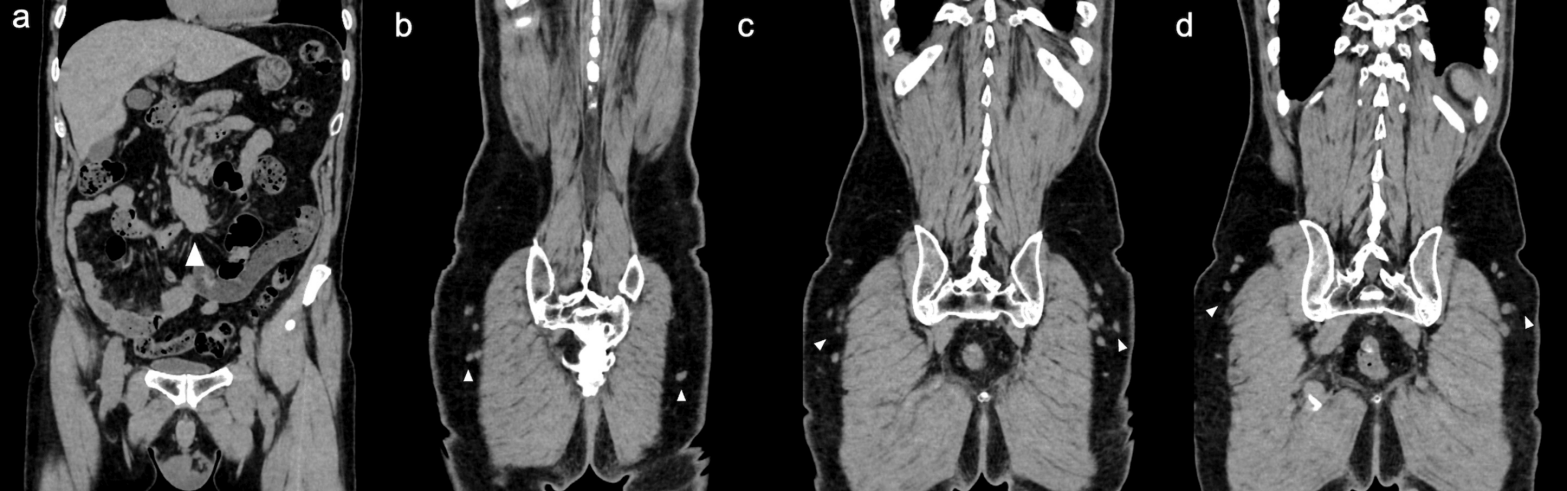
Contrast-enhanced CT images Contrast-enhanced CT images six years after treatment initiation. Coronal view showing no significant changes in primary lesion size (a: arrow) or new lesion development (a). Sequential coronal section images showing persistent lanreotide depots in the buttocks (b, c, d). Persistent nodular lesions (b, c, d: arrows) in subcutaneous nodules on the buttocks over time. CT, computed tomography
